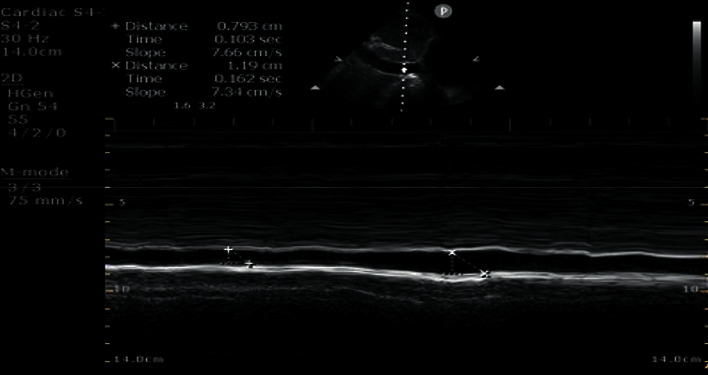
The maximum inferior vena cava diameter (dIVC_max_) was measured as the maximum anterior-posterior dimension at end-expiration using the leading-edge technique (inner edge to inner edge of the vessel wall). The minimum inferior vena cava diameter (dIVC_min_) was measured at end-inspiration. The collapsibility index was calculated using the built-in software available on the ultrasound systems. IVC: inferior vena cava; dIVC_max_: maximum IVC diameter; dIVC_min_: minimum IVC diameter.
